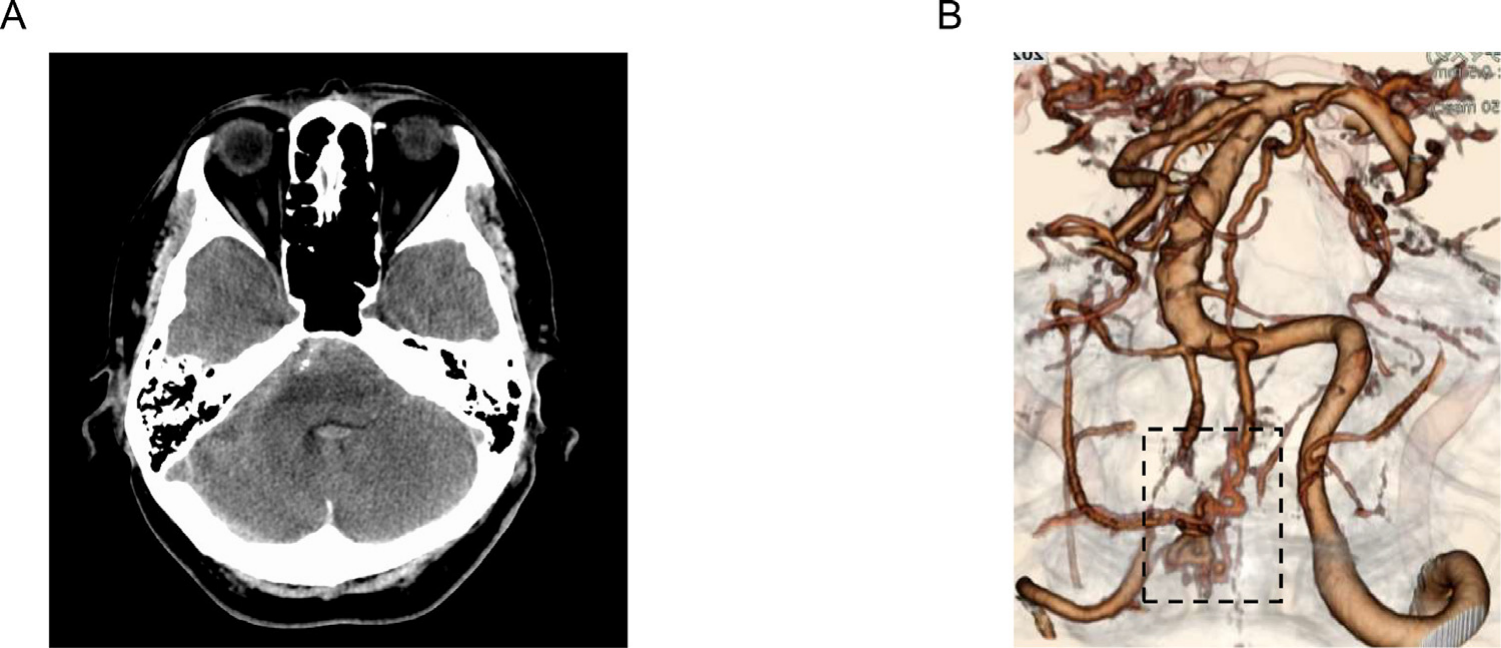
(A) Computed Tomography image shows that the subarachnoid hemorrhage (SAH) is mainly located in the right pontocerebellar cistern. (B) Computed tomography angiography shows abnormal blood vessels around the posterior cranial fossa (area marked by dotted lines).
